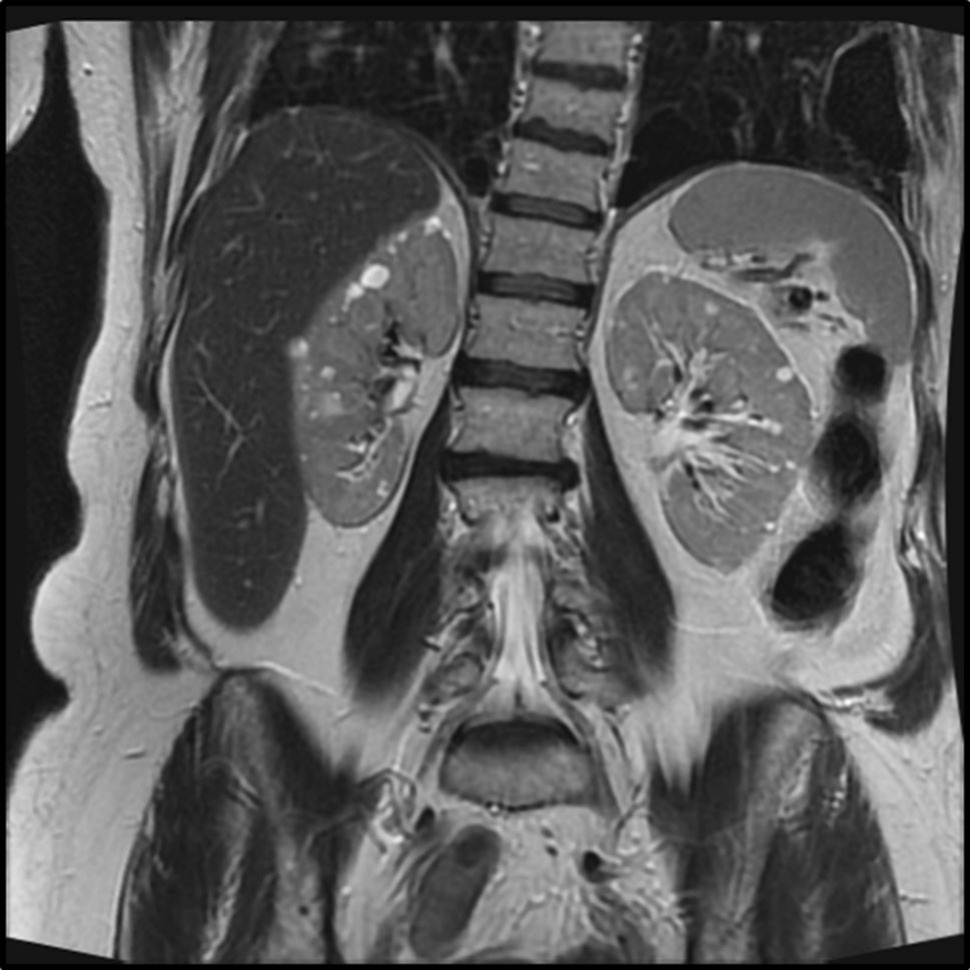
Abdominal magnetic resonance imaging scan of probands showing multiple renal cysts and pulmonary bullae
